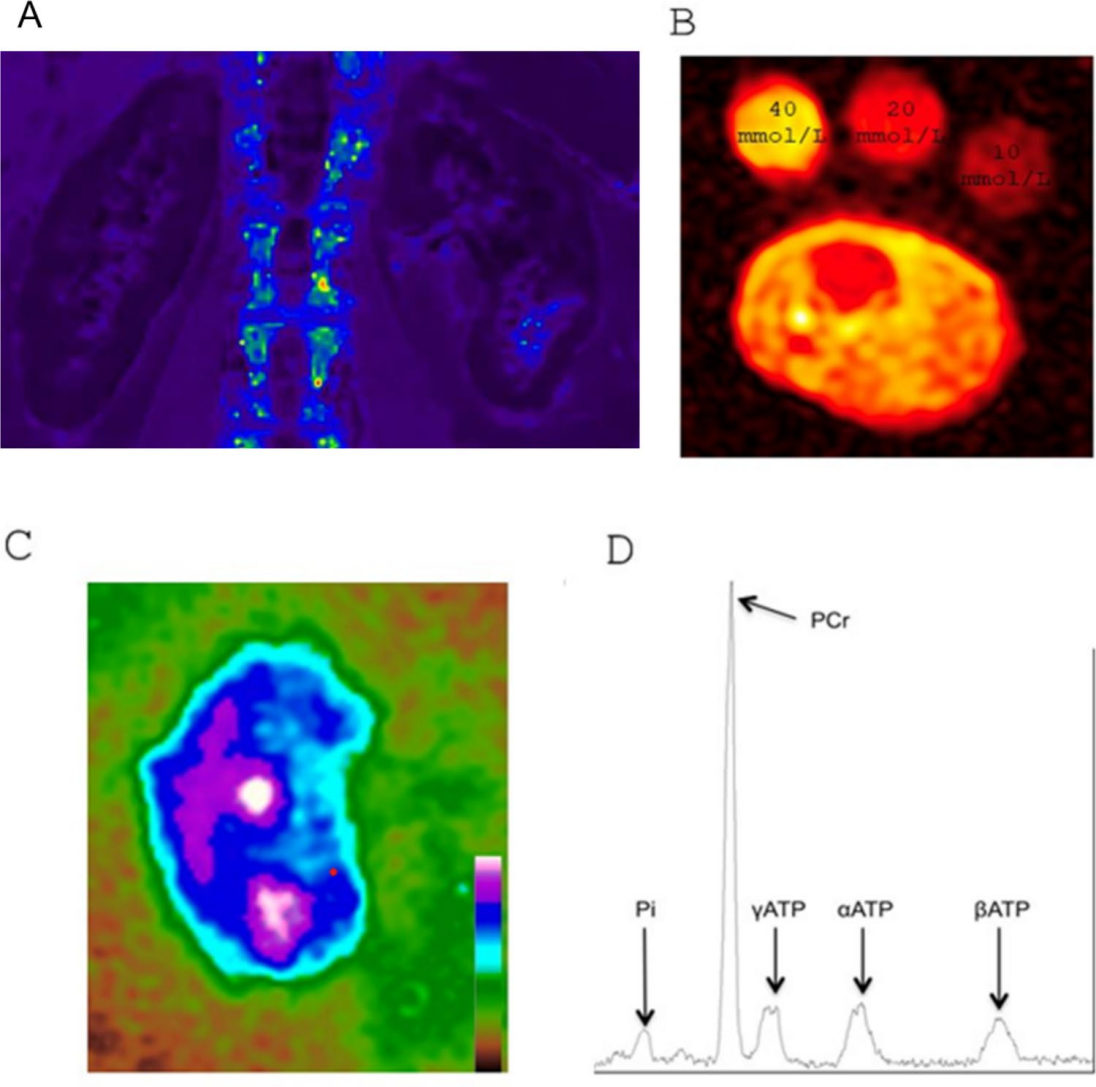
A Images of the kidneys acquired via BOLD-MRI in a healthy subject. B
